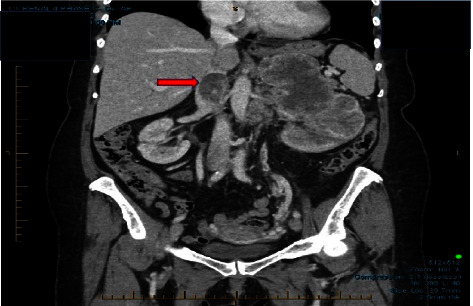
The computed tomography scan coronal images demonstrate tumor thrombus involving the left renal vein extending into the junction of the hepatic/infrahepatic IVC. Arrow indicates tumor thrombus at the junction of the hepatic/infrahepatic IVC.
